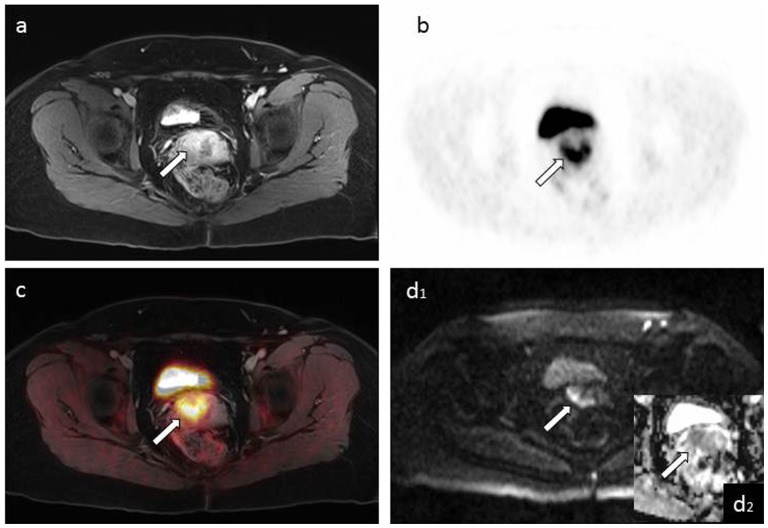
Figure a shows a T1w 3D VIBE image of an inhomogeneously contrast-enhancing mass lesion of the cervix of a 49-year-old patient. Corresponding PET (b) and fused PET/MRI images (c) demonstrate elevated FDG-uptake. The lesion shows corresponding diffusion impairment in DWI (d_1,_ b = 1000) and low signal intensity in the ADC map (zoom image d_2_).
